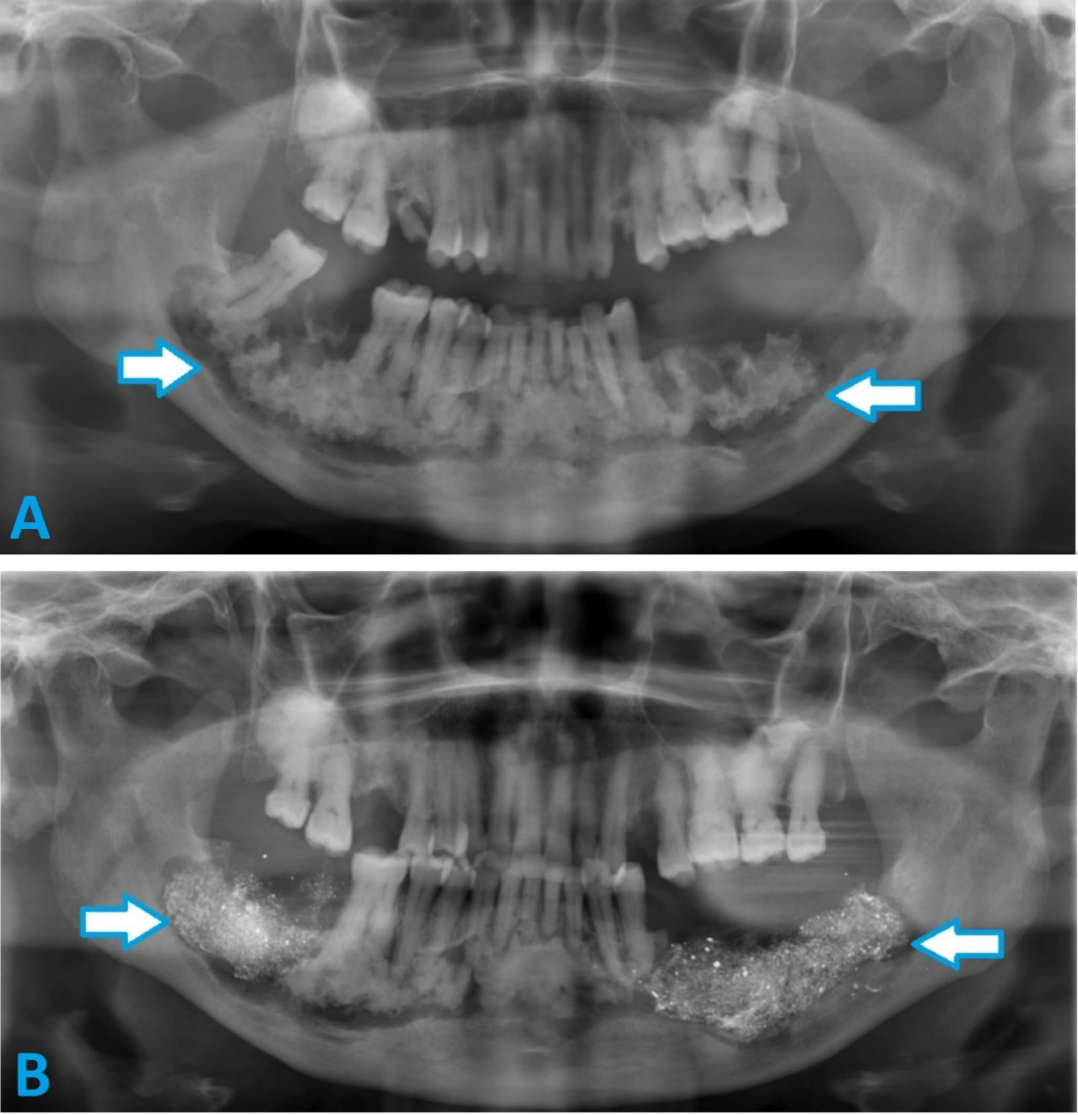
(a) Pre-operative panoramic radiograph of a patient with extensive florid COD and features of osteomyelitis in the mandible bilaterally. (b) Post-operative panoramic radiograph of the same patient post debridement of COD and placement of BIPP impregnated gauze
